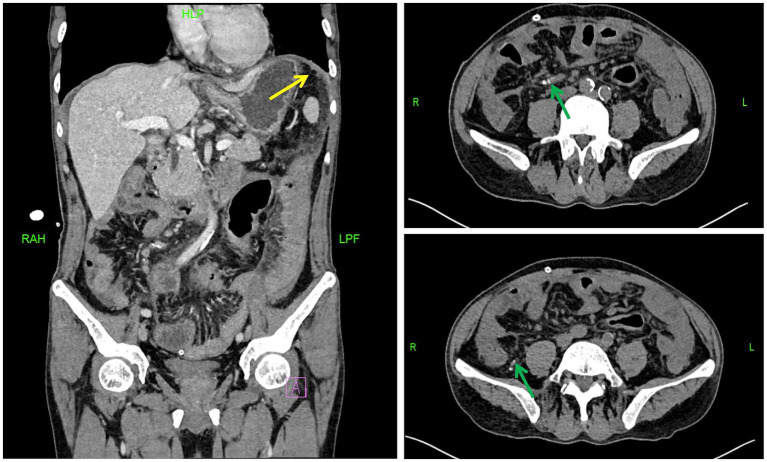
Contrast-enhanced abdominal CT shows thickening and blurring of the omentum around the stomach and transverse colon (yellow arrows), with partial omental calcification (green arrows).
